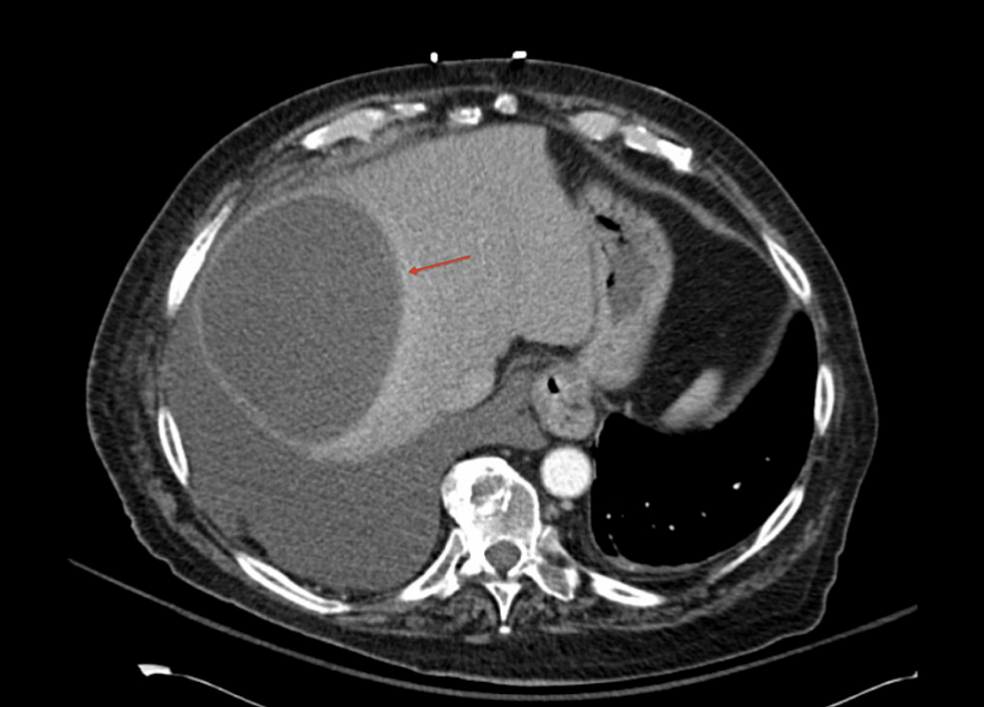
Axial CT with contrast showing 14.2 cm well-defined hypodense lesion in the right liver lobe.
